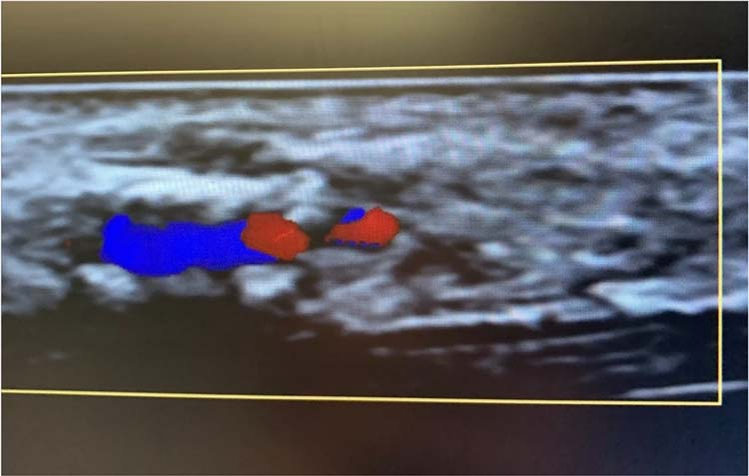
Color flow doppler distinguishing between arterial versus venous flow.
